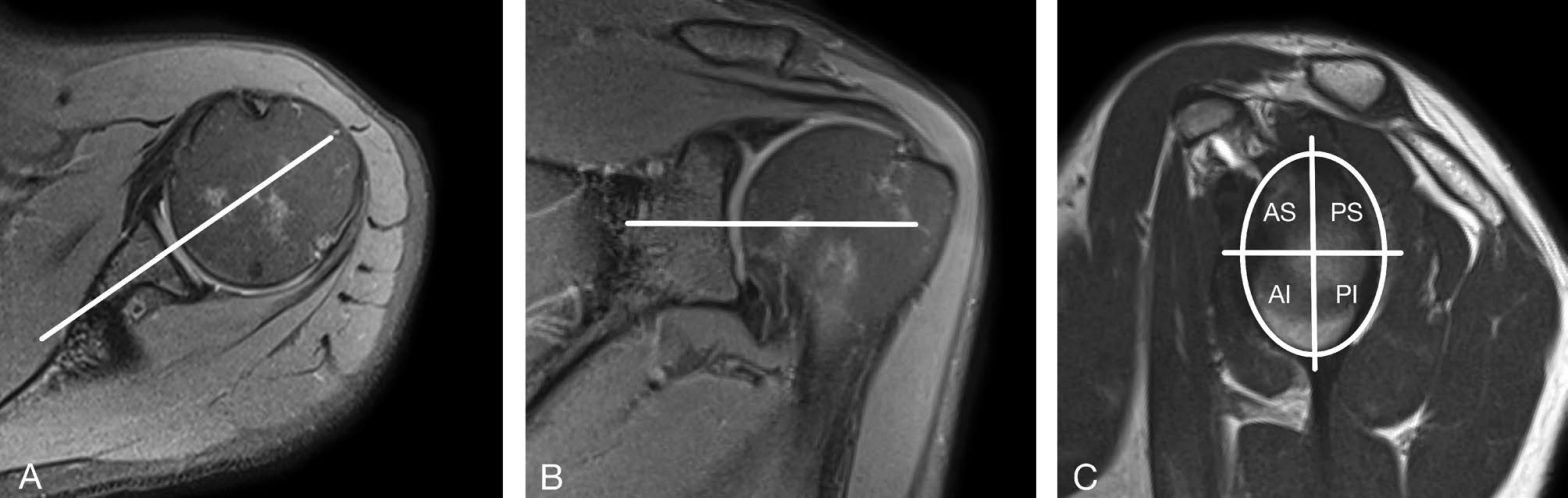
(A) Axial proton-density fat saturated magnetic resonance image used to divide the glenoid cartilage in anterior and posterior segments. (B) Coronal proton-density fat-saturated magnetic image used to divide the glenoid cartilage in superior and inferior segments. (C) Sagittal T2-weighted magnetic resonance image with a depiction of the four-quadrant approach. By halving the glenoid cartilage on axial and coronal images (white lines in A and B) the glenoid cartilage was divided into and anterosuperior (AS), posterosuperior (PS), anteroinferior (AI) and posteroinferior (PI) quadrant.
